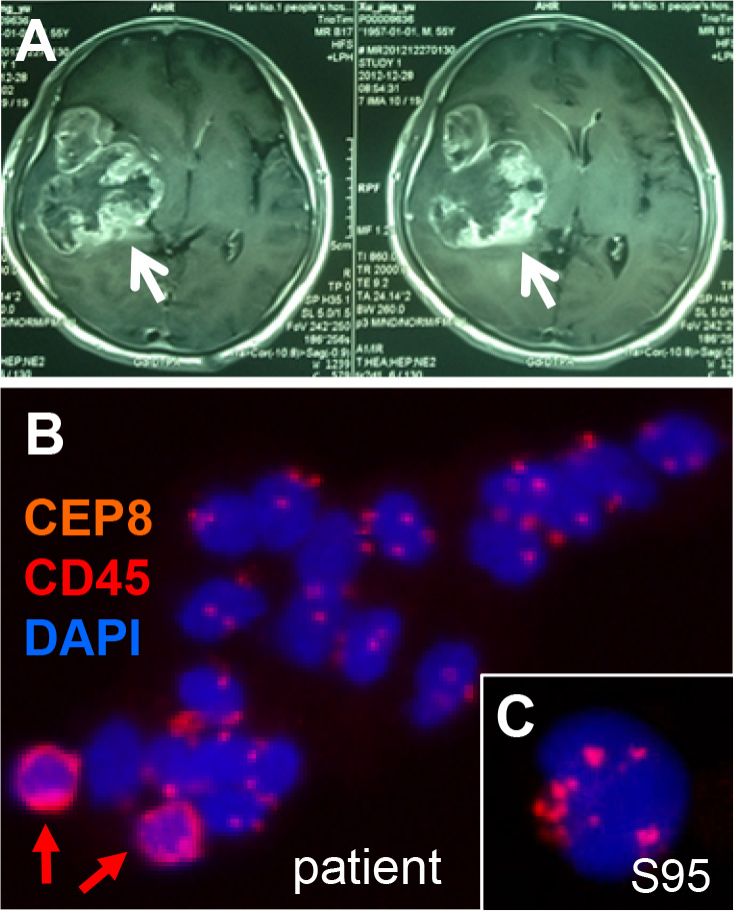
Detection of heteroploid glioma tumor cells disseminated in cerebrospinal fluid of a malignant glioma patient A. MRI scans of the glioma patient. The right temporal glioblastoma with obvious enhancement and necrosis is indicated by arrow. B. A cluster of malignant heteroploid glioma tumor cells (CTM) enriched from cerebrospinal fluid of the same patient is shown. WBCs are indicated by red arrows. C. Image of the cultured primary glioblastoma cells S95 shows heteroploid chromosome 8.
